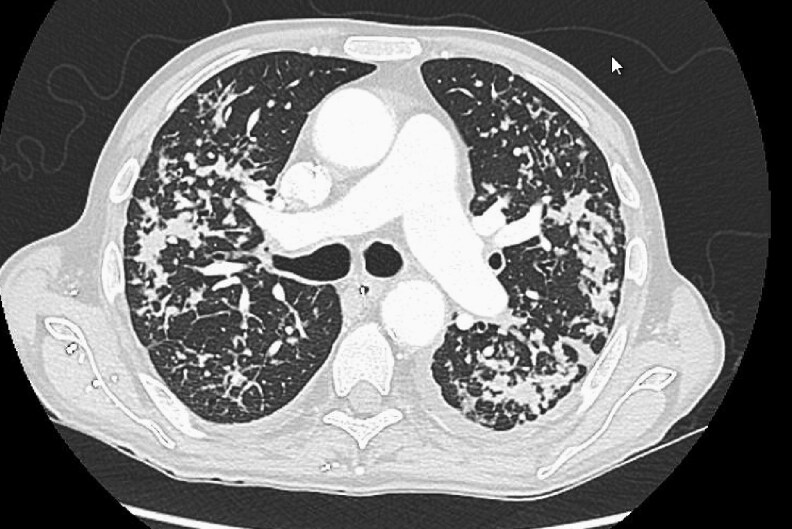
CT scan with a significant pulmonary miliary pattern.
